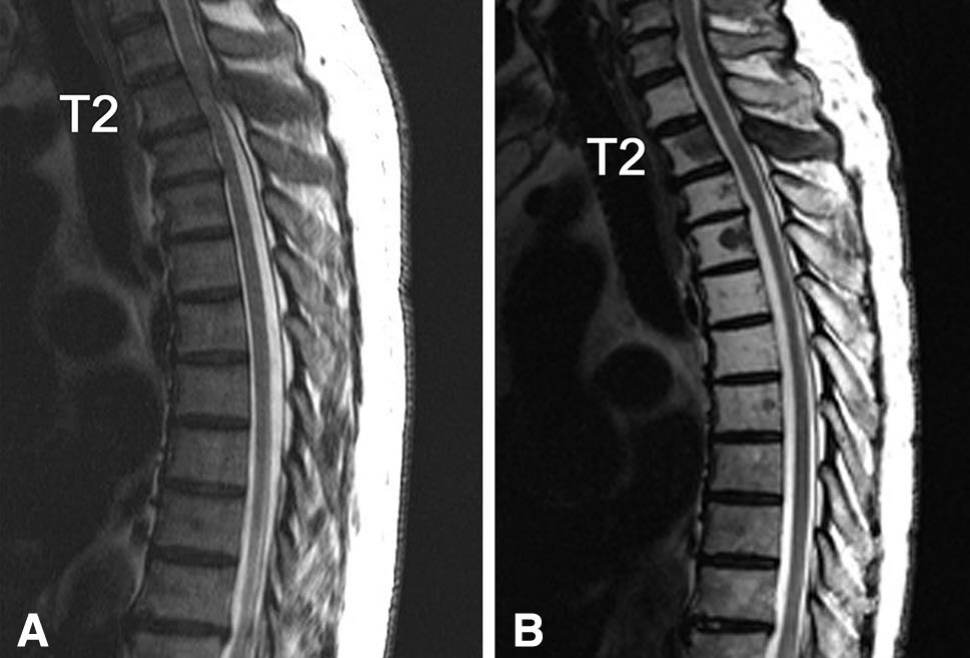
The images of thoracic column in the patients with MSCC of prostate cancer. The tumor in the second thoracic vertebra was improved by radiotherapy. a T2-weighted sagittal MRI images before the radiotherapy. b T2-weighted sagittal MRI images 1 year after the radiotherapy
